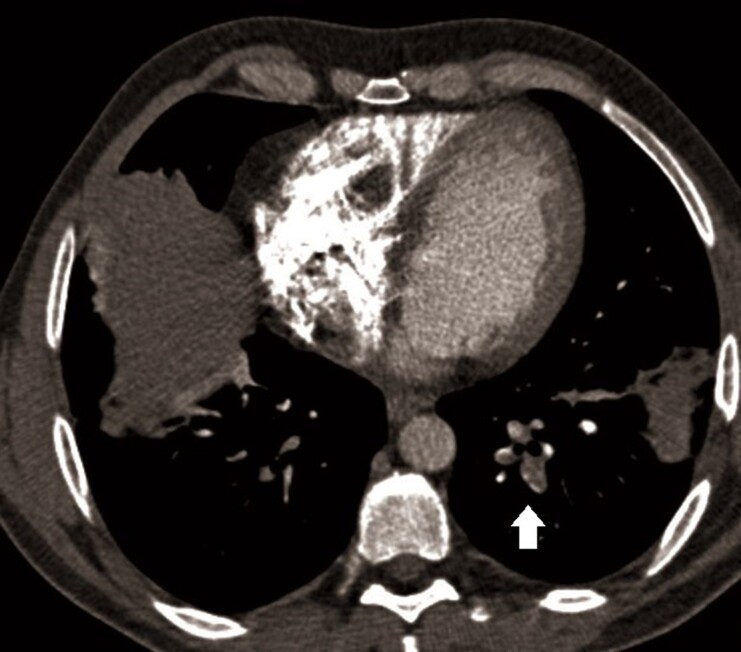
Thorax CT scan with iodinated contrast, axial view. The white arrow indicates PE in the left lower lobe posterior basal sub-segmental pulmonary artery.
